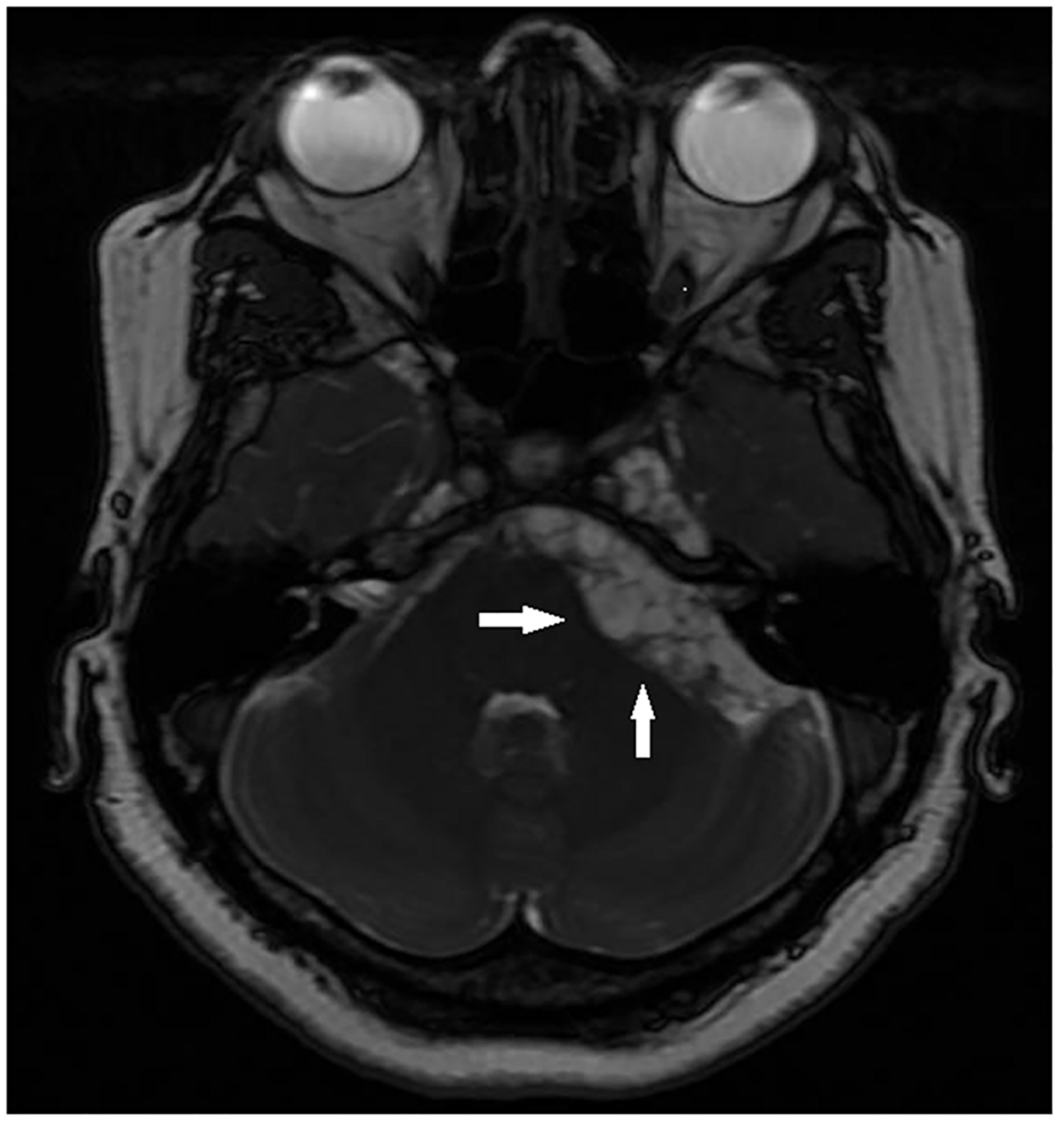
Multiple cysticerci located in the left pontocerebellar cistern (MRI FIESTA).
